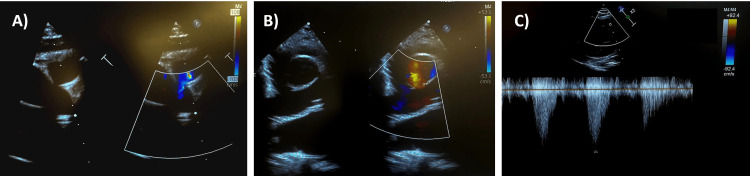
Cardiac echocardiography (A) Ventricular septal defect; (B) Tricuspid atresia; (C) Glenn shunt
